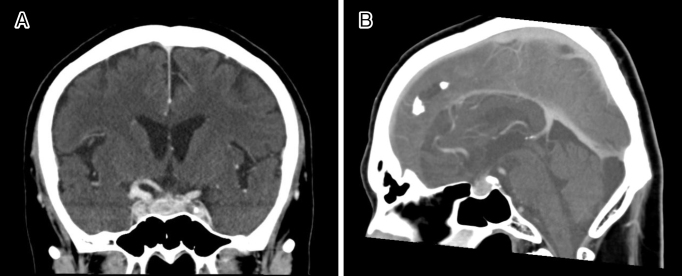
A. Contrast-enhanced coronal computed tomography (CT) of the head displaying an enlarged pituitary gland and stalk. An aneurysm of the right internal carotid artery is suspected. B. Contrast-enhanced sagittal CT of the head displaying an enlargement of the pituitary gland and stalk.
